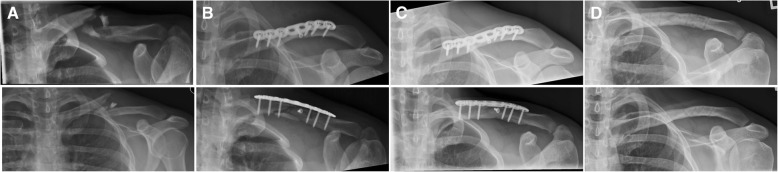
a X-ray in two planes of a 25 years old male patient with multifragmentary midshaft clavicular fracture, b postoperatively, c 5 months after surgery with consolidation of the fracture and d 6 weeks follow-up after hardware removal done 12 months after initial surgery shows solid bone union
